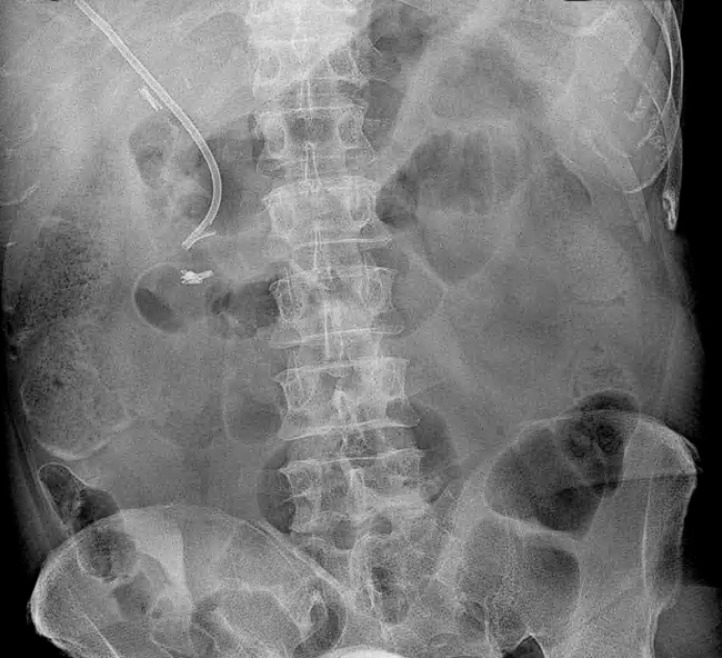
Abdominal radiograph taken after 12 hours showing no signs of perforation.
